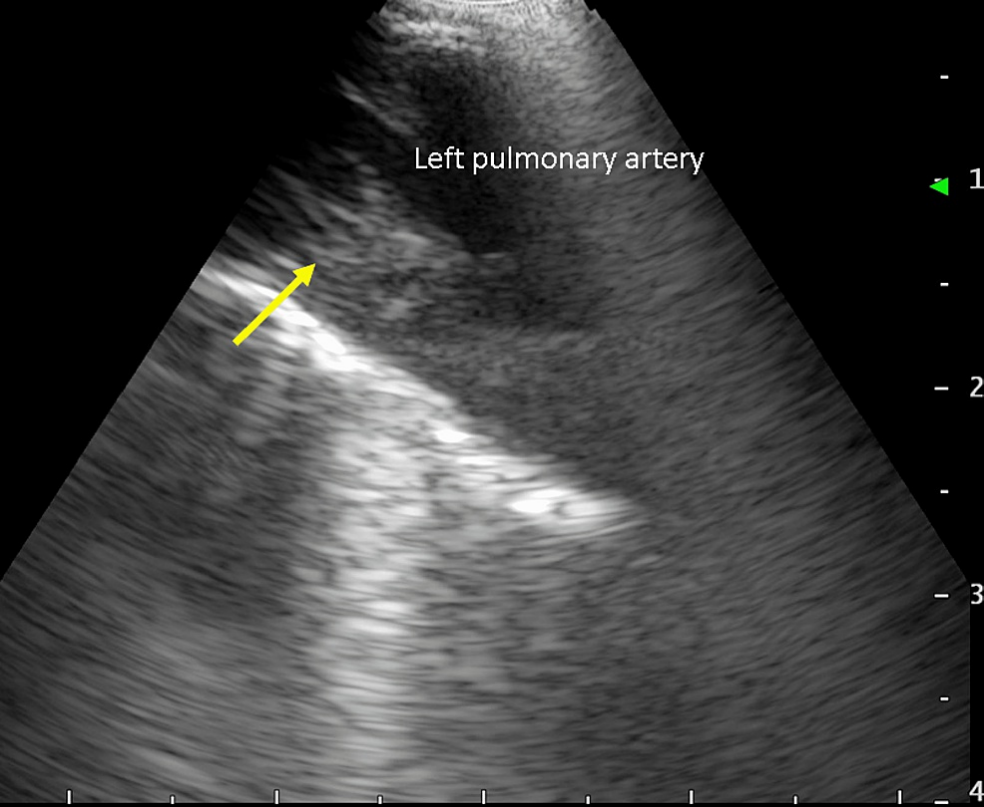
EBUS showing a thrombus in the left pulmonary artery (yellow arrow). EBUS: endobronchial ultrasound
